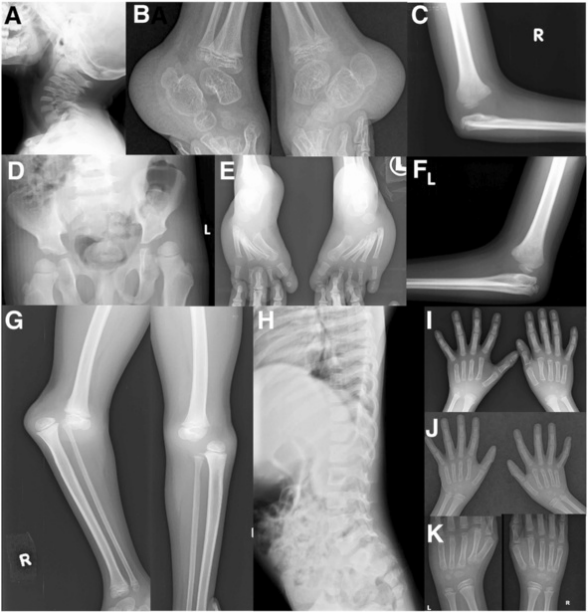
Radiographs of patient 4. He had normal cervical spine (a), double ossification of calcaneum in both feet (b), bilateral elbow dislocations (c and f), normal hip joint (d), bilateral metatarsus adductus (e), bilateral knee dislocations (g), normal thoracolumber spine (h), delayed carpal ossification at 4 years (i), 5 years (j), and 6 years (k) of age as well as abnormal distal phalanx of thumbs and small distal phalanges (j)
